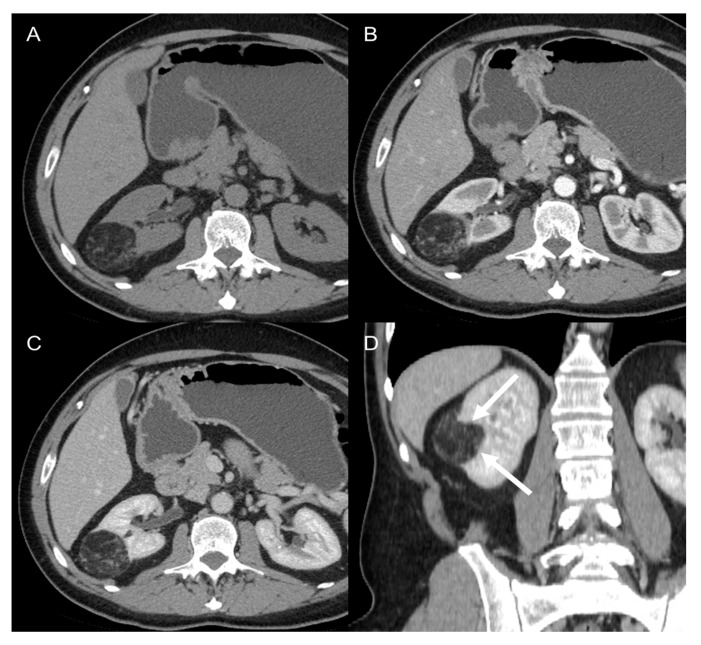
Renal angiomyolipoma in 62-year-old man. Axial CT images on the pre-contrast (A), arterial (B), and venous (C) phases and coronal venous (D) phase show a 4.5 cm heterogenous adipose lesion deforming the edge of the right kidney with a “beak” shape appearance (claw sign, arrows).
